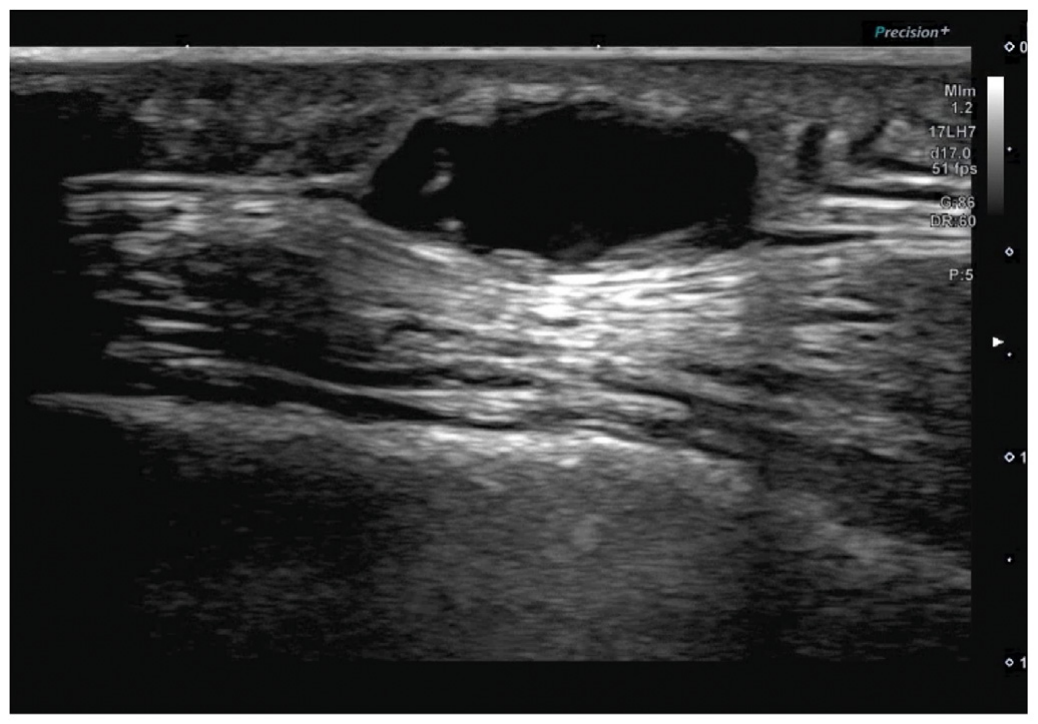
Aneurysm visualized on ultrasound.
